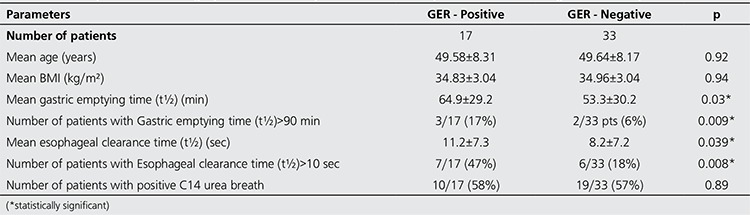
A comparison of scintigraphic and clinical parameters of GER positive and GER negative obese patients
